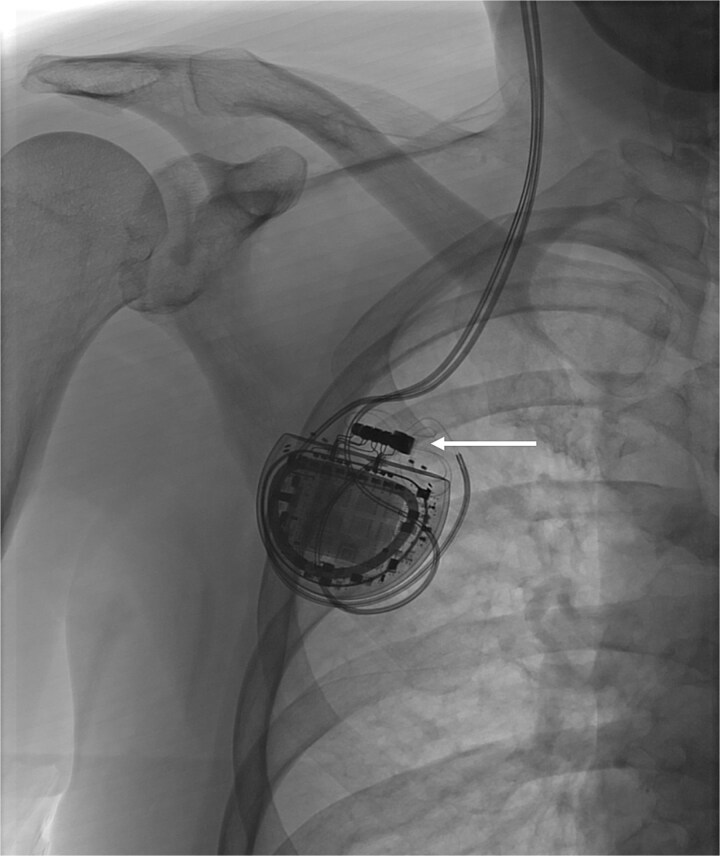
Postoperative chest radiograph following surgical revision demonstrating restoration of normal r-IPG orientation after repositioning and secure fascial anchoring. The coil-receiving surface has been returned to an anterior orientation and the leads exit the device from the left side (white solid arrow). No lead displacement or hardware abnormality is identified.
